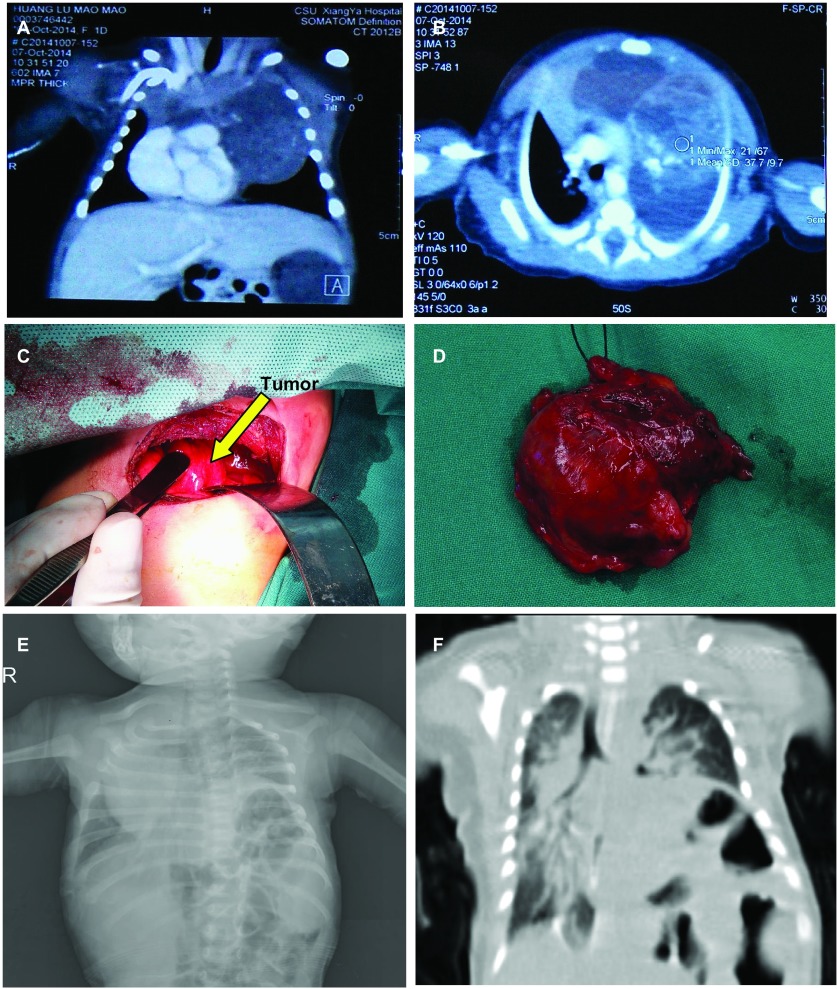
患者影像学图片。A、B为术前患儿CT，可见前纵隔巨大占位，纵隔明显右移，肿瘤内密度不均匀；C为术中图片，D为完整切除的肿瘤；E、F为术后复查的胸片和CT，可见纵膈仍然向右移位，左侧膈肌明显抬高。 Pictures of patient. A and B are preoperative CT scan, which show huge anterior mediastial tumor with different Hu on CT and mediastium shifts to the right. C is in operation and D is the tumor removed completely. E and F are the postoperative X-ray and CT, from which mediastium still shifts to right and left diaphragm rises up obviously. CT: computed tomography.
